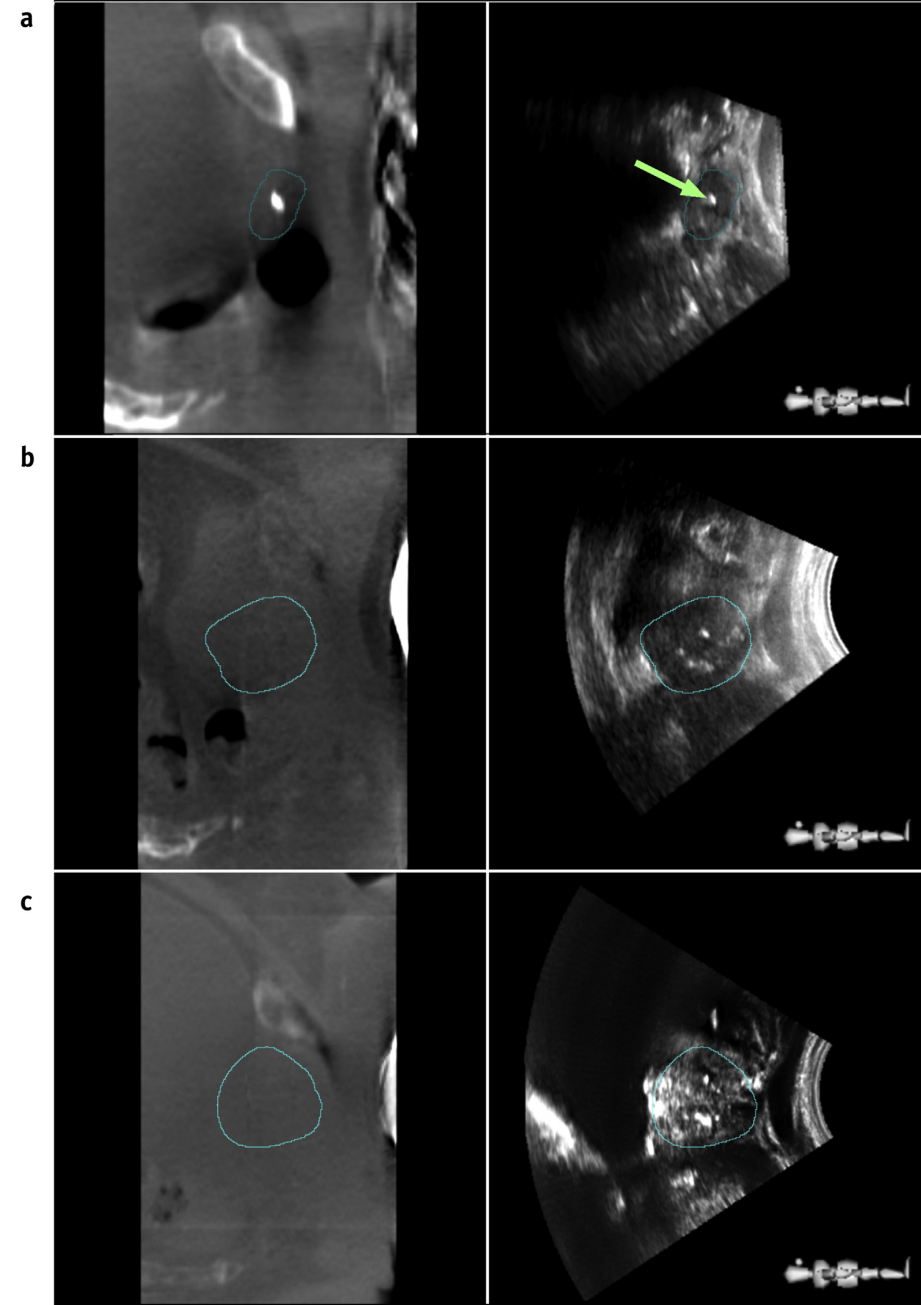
a, Sagittal cone beam computed tomography image with associated transperineal ultrasound image depicting prostate guidance positioning volume contour (blue) and fiducial marker identified visually (green arrow). b, c, Guidance positioning volume features visible on transperineal ultrasound images but not cone beam computed tomography images. High-intensity (white) ultrasound features seen in the central region of the prostate likely are calcifications. Ultrasound features are also observed near the urethra and bladder-prostate interface. (A color version of this figure is available at https://doi.org/10.1016/j.ijrobp.2018.04.008.)
